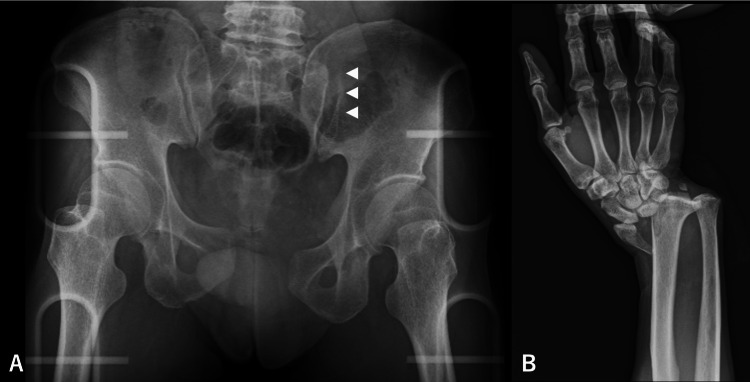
Preoperative X-ray (A) Disarticulation of the left sacroiliac joint (△) and the pubic symphysis. (B) Dislocated fracture of the right wrist joint.
